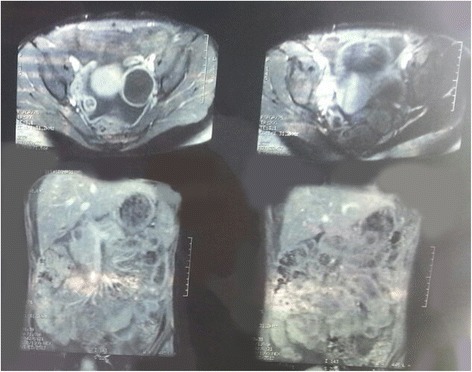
Abdominal MRI: Cystic formation in left tubo-ovarial complex
